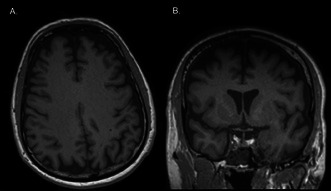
FIGURE 1 Axial (A) and coronal (B) T1‐weighted brain MRI demonstrating frontal‐predominant cortical atrophy.
